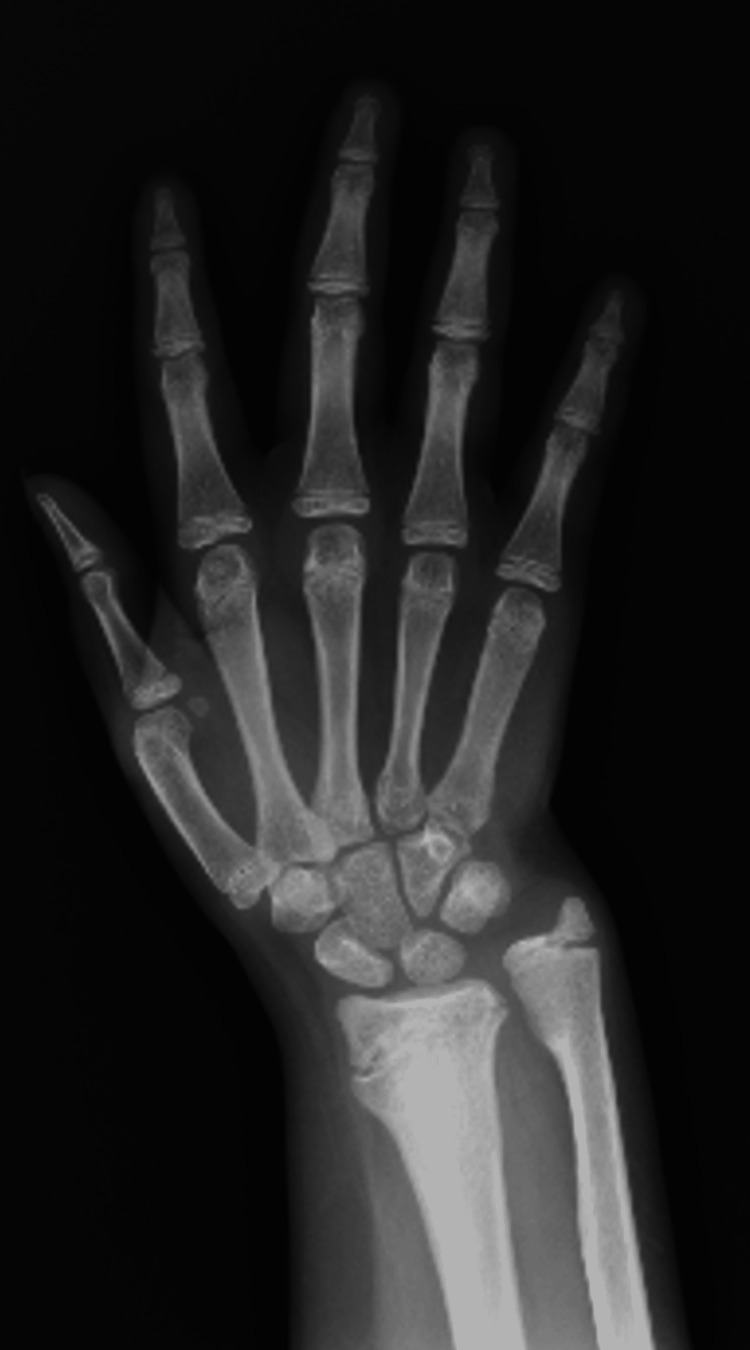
Wrist and hand X-ray for bone age.
